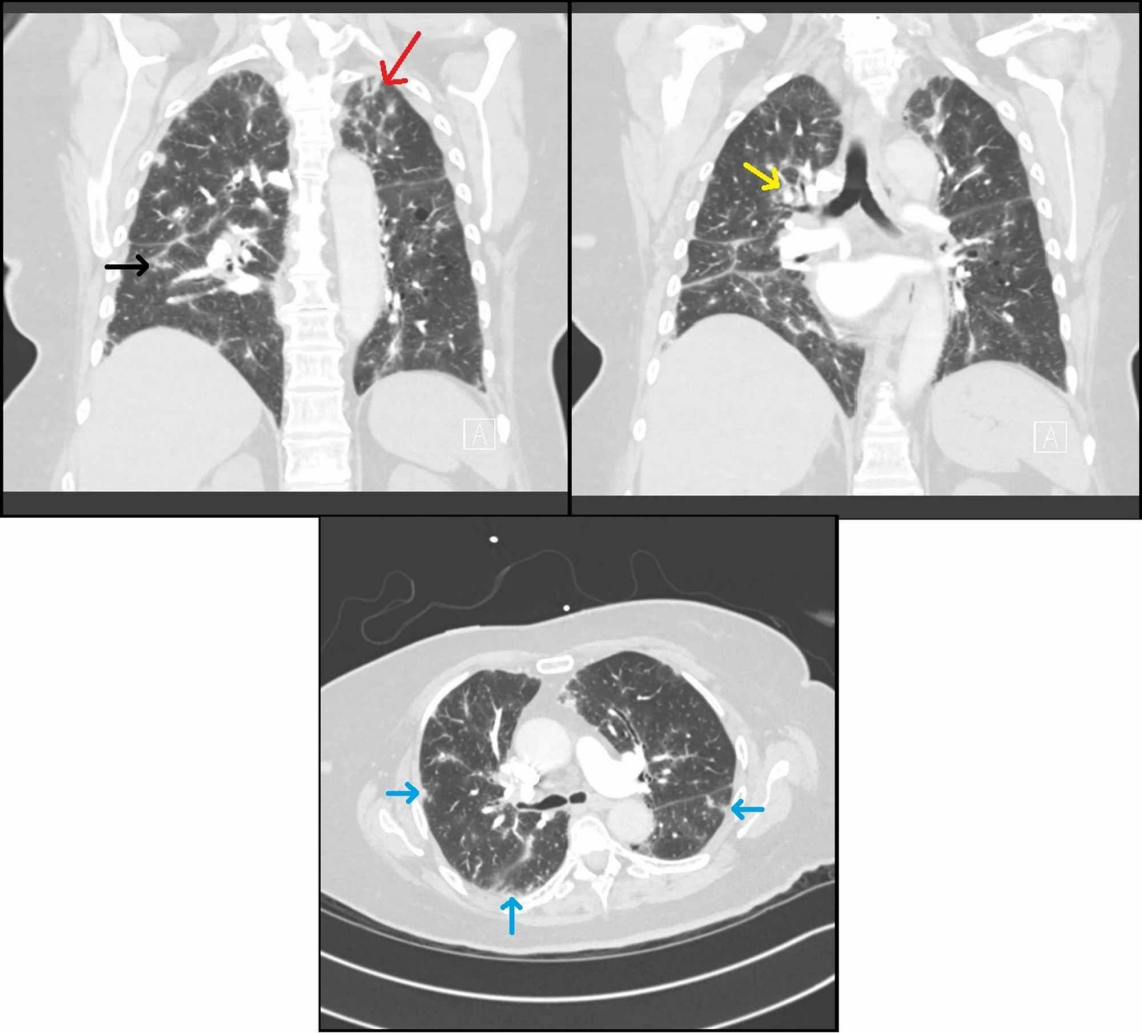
CT imaging on presentation A) Coronal CT of the chest noting subpleural ground glass opacities (black arrow) and slight upper lobe predominance of ground glass opacities (red arrow). B) Coronal CT of chest noting peribronchovascular ground glass opacities (yellow arrow). C) Axial CT of chest demonstrating diffuse nodular ground glass opacities in a subpleural (blue arrows) distribution.
